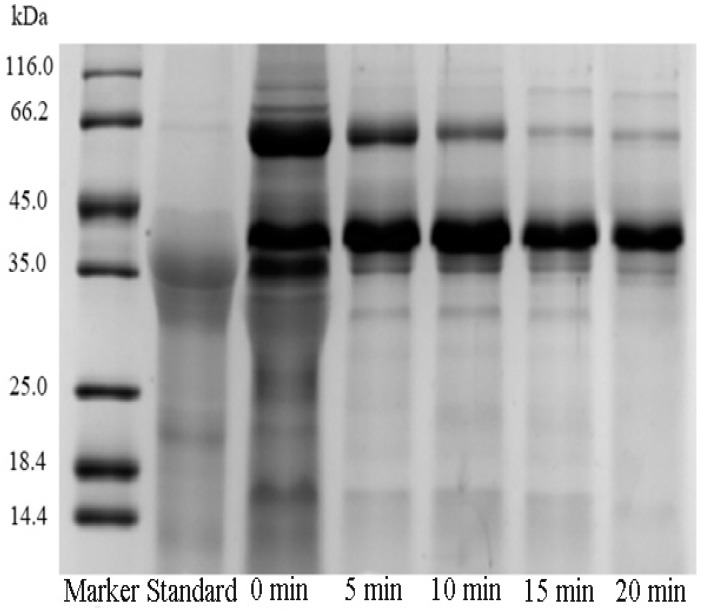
SDS-PAGE of Pv for different heating times (0, 5, 10, 15, and 20 min). Other operating conditions: Heating temperature, 80 °C; ultrasound power, 600 W; and ultrasound time, 10 min.
